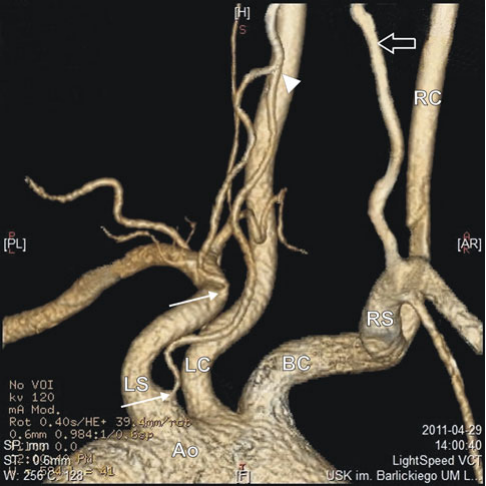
Three-dimensional CT reconstruction of the arteries. *White arrows* origins of the duplicated left vertebral arteries, *black arrow* right vertebral artery, *arrowhead* level of fusion of duplicated left vertebral arteries, *Ao* aortic arch, *BC* brachiocephalic trunk, *LC* left common carotid artery, *LS* left subclavian artery, *RC* right common carotid artery, *RS* right subclavian artery
